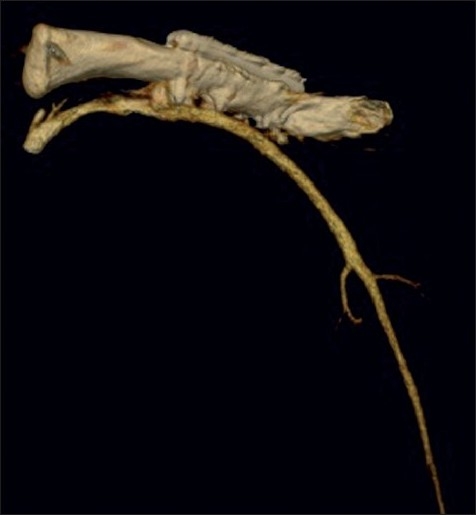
CT arteriogram of left clavicle, demonstrating penetration of the axillary artery by the prominent medial screw with associated pseudoaneurysm of the axillary artery. All structures apart from the clavicle and subclavian and axillary arteries have been subtracted
